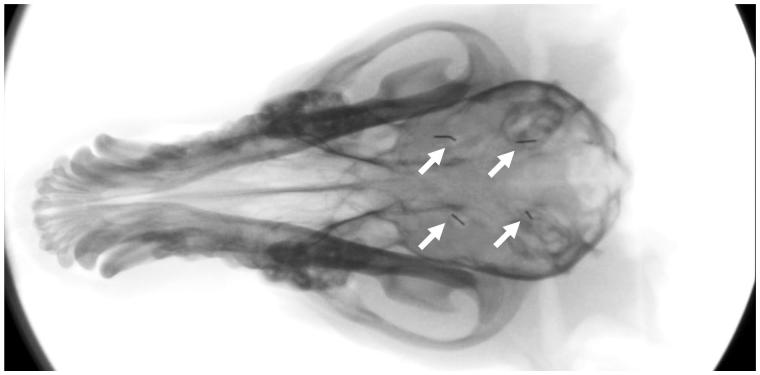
The four locations where hyperthermia was applied are indicated by fiducial markers (white arrows) in this X-ray image. The hyperthermia probe was placed through burr holes, penetrating ∼10–15 mm deep into brain tissue.
